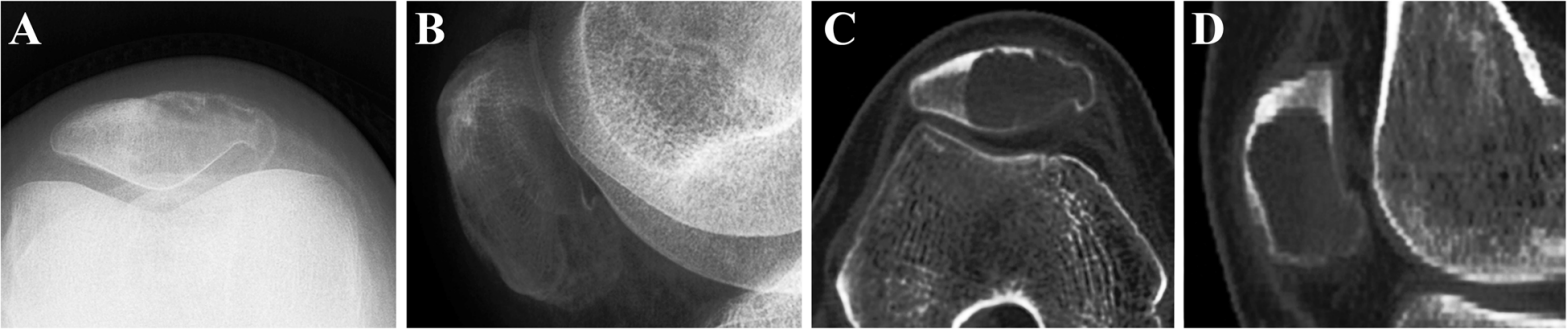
Patellar giant cell tumor. (A, B) An enlargement of the cystic lesion in the patella and a bone translucency with peripheral rim change can be seen at the lateral down part of the patella on radiographs. (C, D) CT indicates that there is a well-defined lytic lesion with the thin cortex occupying two-thirds of the patella.
